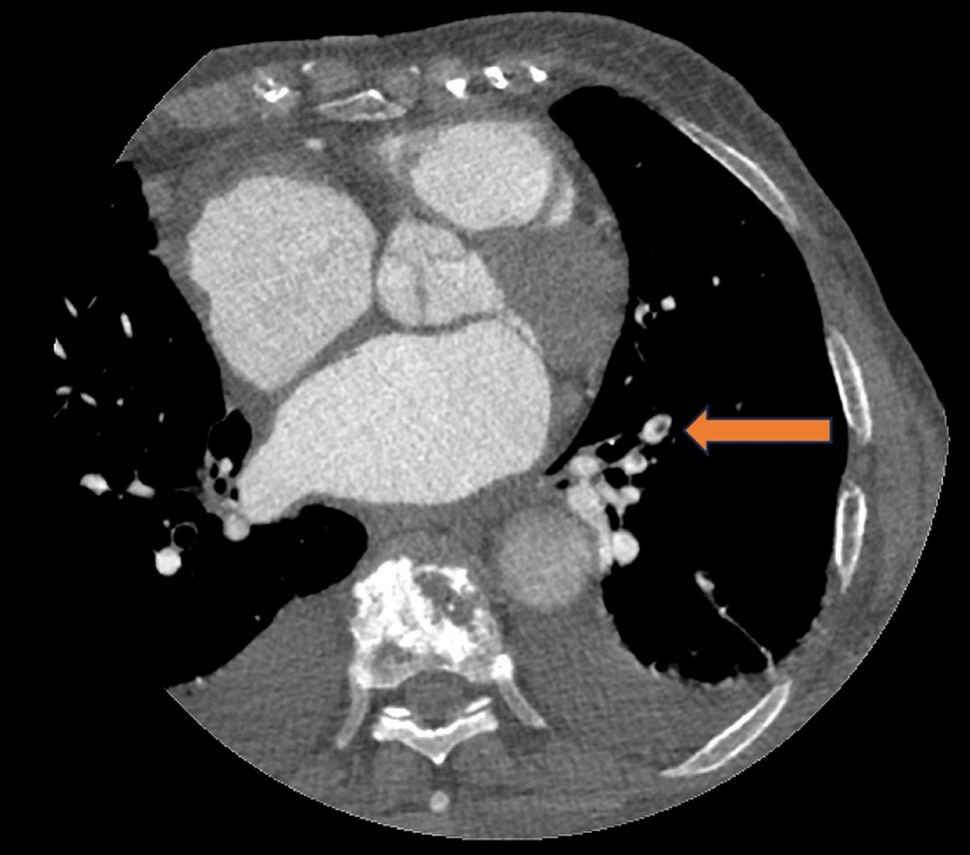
Left lower lobe subsegmental pulmonary embolism (PE) on a CTA targeting the left atrial appendage of an 88-year-old female patient. The PE was not reported in the initial radiology report, but was subsequently identified by the AI algorithm
